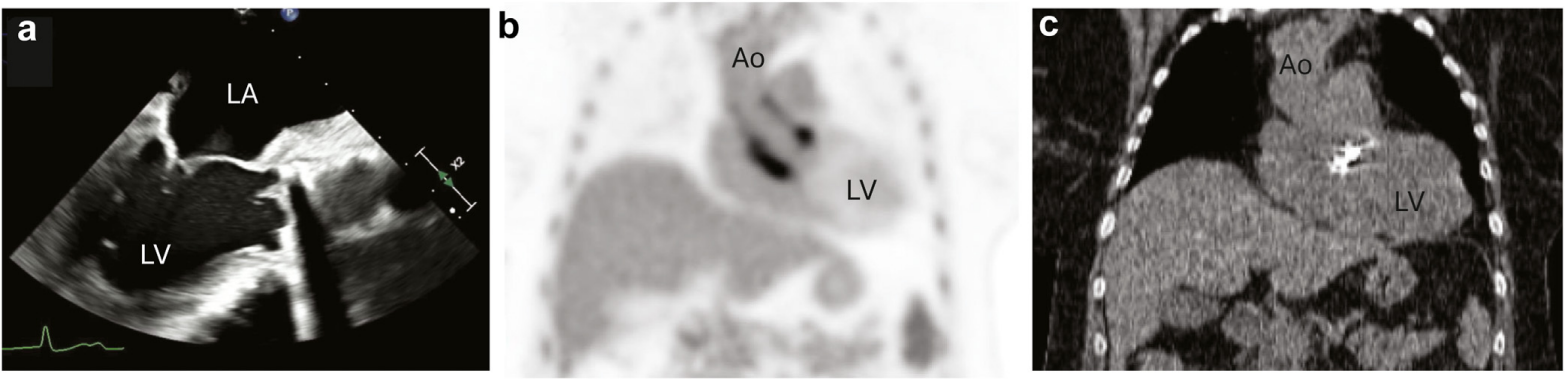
Patient with infective endocarditis of post-Bentall with a mechanical valve. (a) Transesophageal echocardiographic image during diastole demonstrating vegetations on the mechanical valve in the aortic position and a thickened aortic root and ascending aorta. (b) Fludeoxyglucose F18 positron emission tomography (FDG-PET) demonstrating activity involving the mechanical aortic valve and aortic root. (c) The matching cardiac computed tomography image demonstrating the location of the mechanical aortic valve. Abbreviations: Ao, aorta; LA, left atrium; LV, left ventricle.
